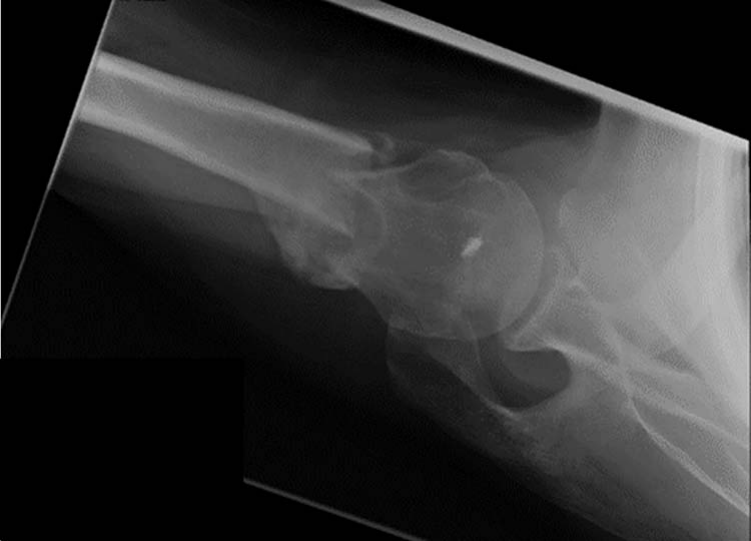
Axial view X-ray taken 14 weeks post-injury showing excellent fracture union and articulation between the humeral head and glenoid.
